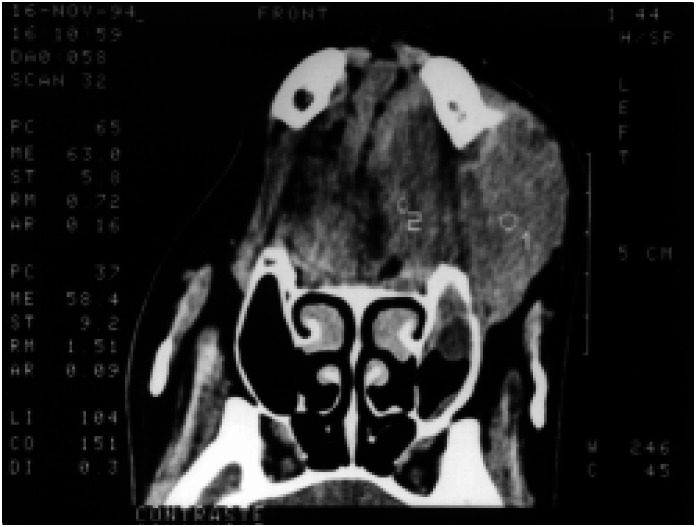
CT scan showing an expansive lesion on the left portion of the mandible, causing deviation of the tongue away from the midline.
